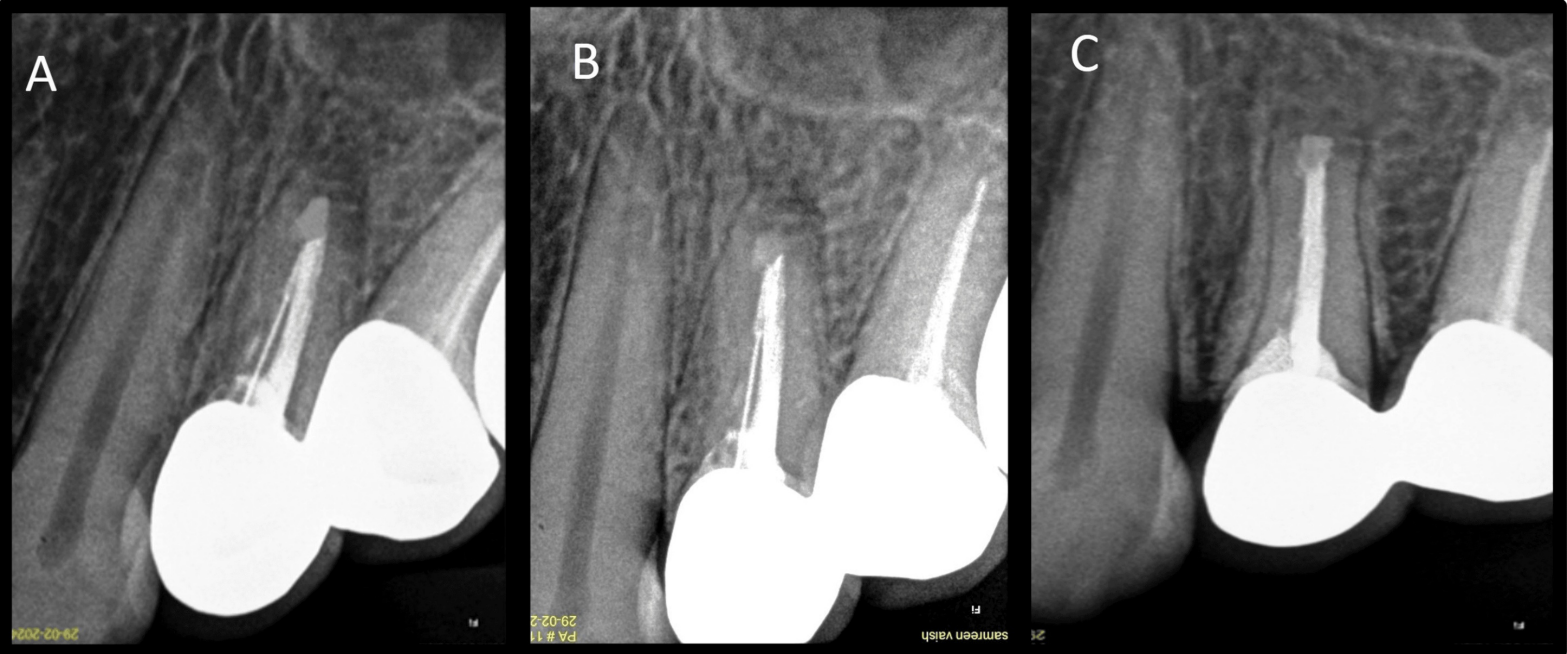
Follow-up images demonstrating radiographic bone fill A: Three-month follow-up, B: Six-month follow-up, C: One-year follow-up
